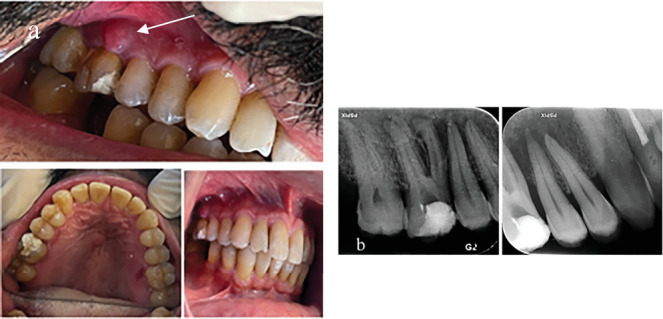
(a) Clinical images of the posterior maxillary quadrant; the arrow shows the swelling and fistula formation around the first molar. (b) Periapical radiographs showing bone destruction around the maxillary first molar and premolar teeth.
